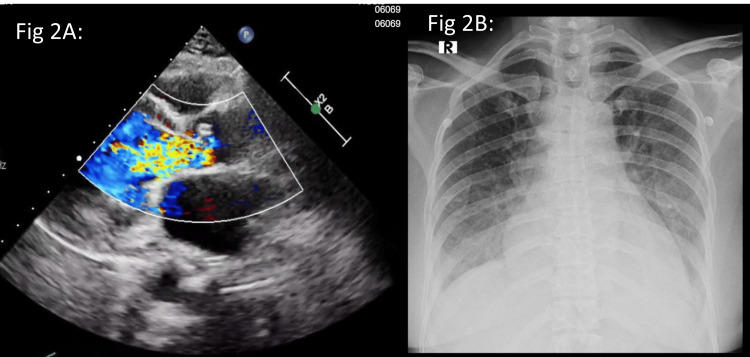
Case 2: Transthoracic echo and chest X-ray Transthoracic echo (2A) colour Doppler image showing flow turbulence across the aortic valve, suggestive of aortic regurgitation. Chest X-ray (2B) showing cardiomegaly with pulmonary venous congestion.
